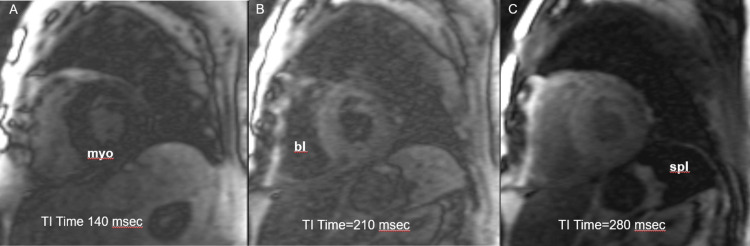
Cardiac magnetic resonance imaging Delayed post-contrast TI scout images show abnormal inversion times with a Type IV nulling pattern. The myocardium (myo) nulls before the blood pool at 140ms (A), the blood pool (bl) nulls later at 210ms (B) and splenic nulling (spl) occurs at 280 ms without coinciding with myocardial nulling (C).
